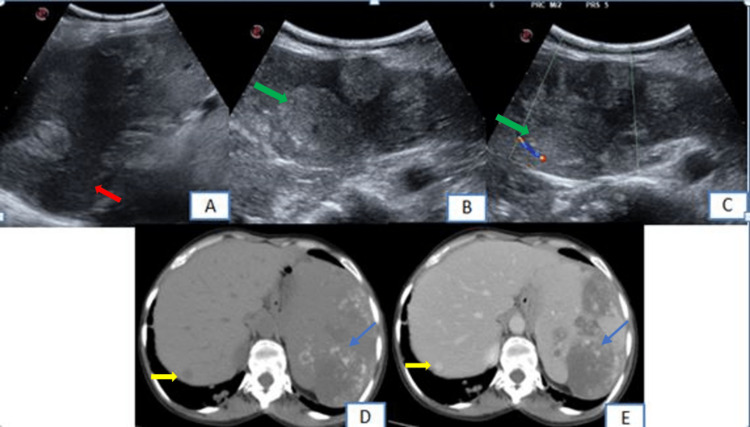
Abdominal US (plain) images and CT images showing splenic and hepatic lesions Abdominal US (plain) images (2A, 2B, 2C) showing gross splenomegaly (red arrow), with multiple, well-defined, heterogeneously hyperechoic lesions of varying sizes scattered throughout the splenic parenchyma, with no increased vascularity on Doppler evaluation (green arrow). Plain (2D) and contrast-enhanced (2E) CT images show multiple minimally enhancing lesions in the spleen (blue arrow). Hypo-dense enhancing nodule is noted in the right lobe of the liver (yellow arrow).
